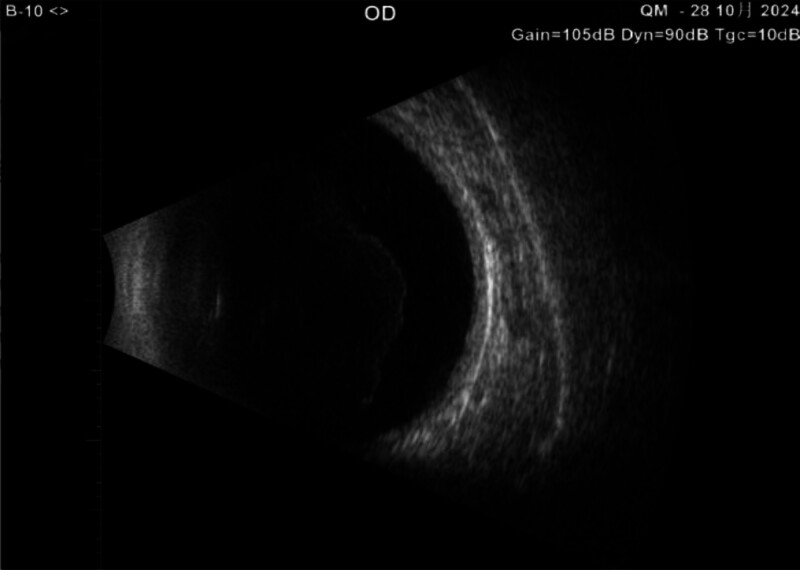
Ophthalmologic ultrasound: vitreous opacity in the right eye and posterior vitreous detachment in the right eye.
